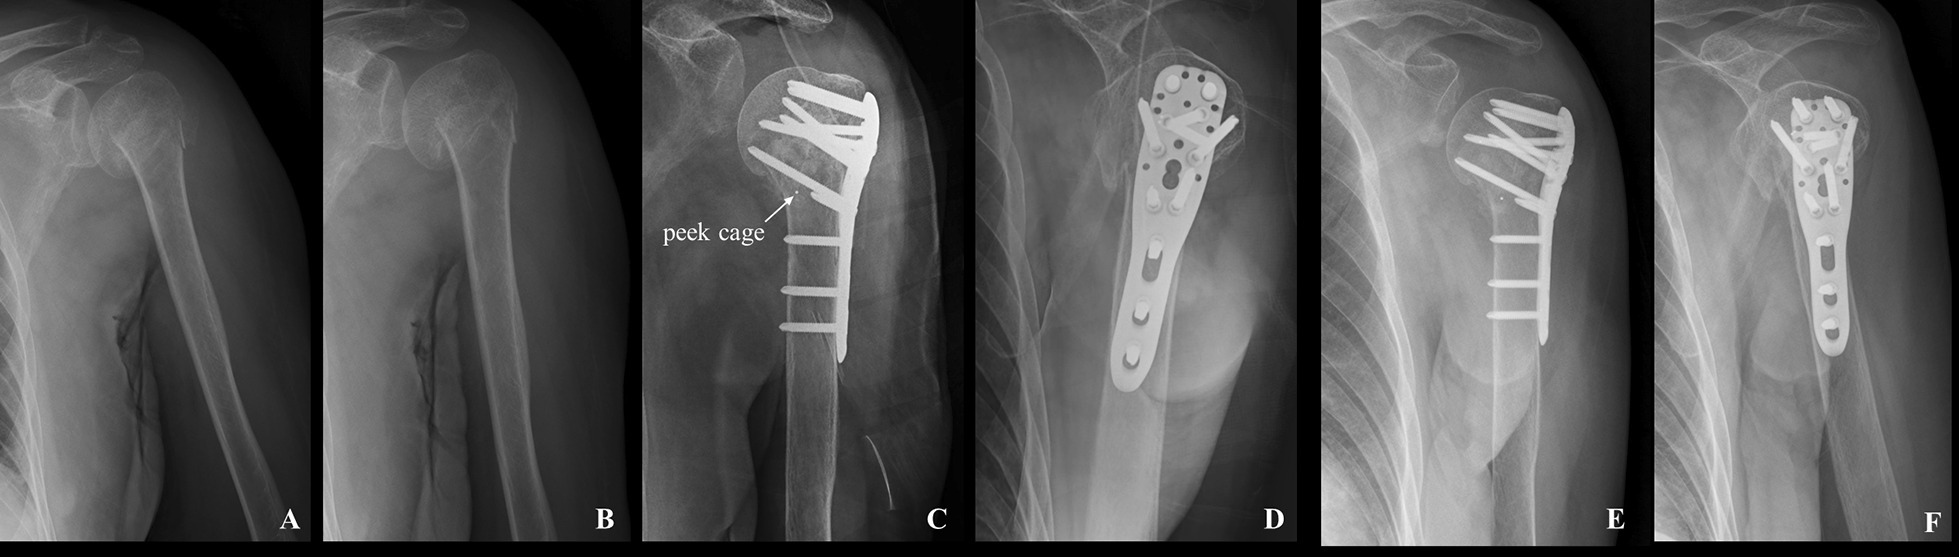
Radiograph of a 47-year-old woman with 3-part fractures. a, b Preoperative X-ray film; b, c Peek cage was used as volumetric filling in the medial site to prevent the humeral head collapse, facilitating reduction, and to provide mechanical support; e, f X-ray film 3 months after the operation
